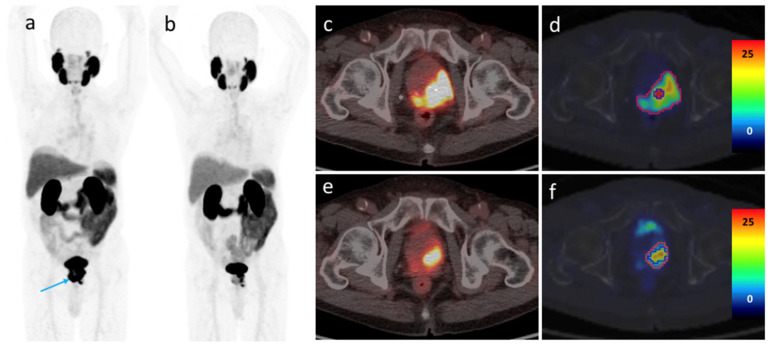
An 85-year-old patient with prostate cancer (clinical stage IVB, PSA 84.15 ng/mL, Gleason score 5 + 4). (a) A maximum intensity projection obtained before treatment revealed the prostate tumor (blue arrow) and a focal lesion beneath the primary lesion (pubic bone metastasis). (b) A second scan after three months of goserelin therapy showed a significantly decreased tumor metabolic volume. (c) and (d) Different tumor segmentation methods (threshold method: red contour; 45% SUVmax method: blue contour) yielded varying results. PSMA-TV and PSMA-TL values were 4.52 and 190.39, respectively (45% SUVmax method) and 53.59 and 824.69, respectively (threshold method). (e) and (f) After androgen deprivation therapy, PSMA-TV and PSMA-TL values were 3.14 and 44.87, respectively (45% SUVmax method) and 14.53 and 119.36, respectively (threshold method).
